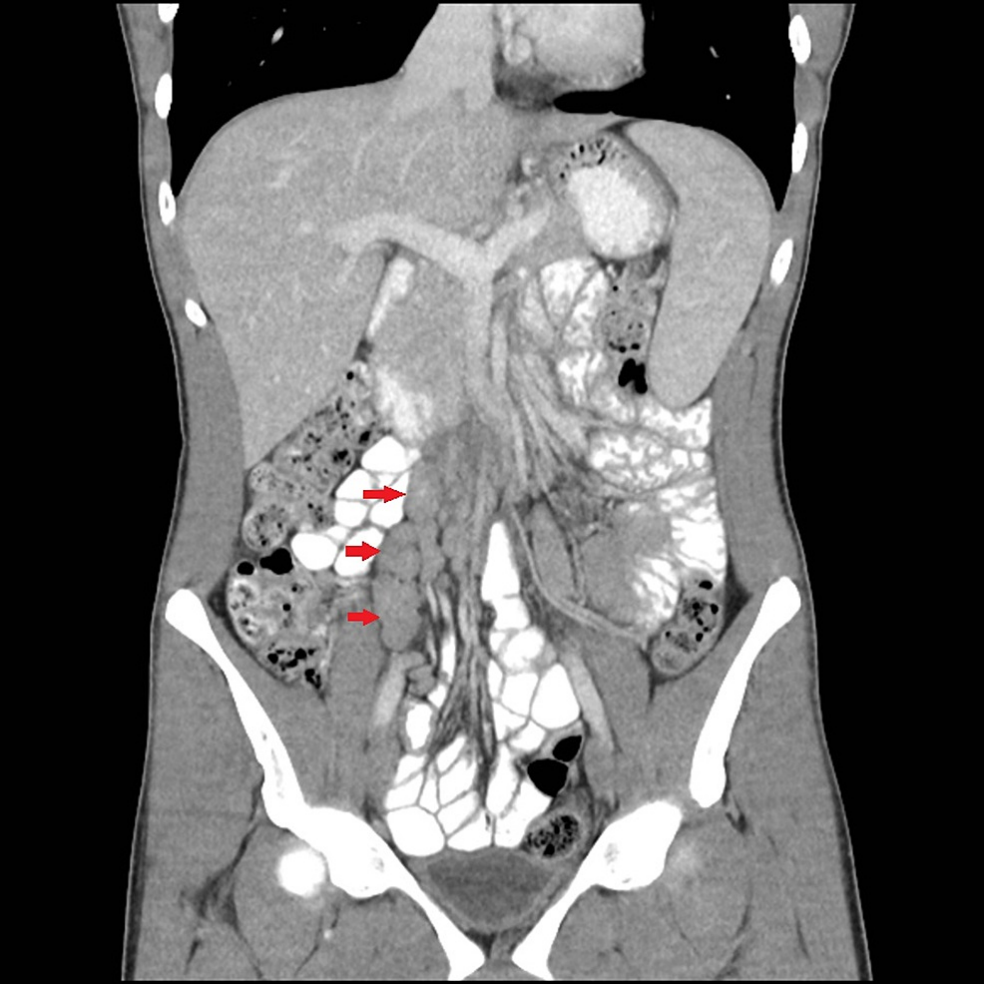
Coronal reformatted image from CT of the abdomen and pelvis with oral and IV contrast demonstrates a cluster of enlarged mesenteric lymph nodes in the right lower quadrant (red arrows).
